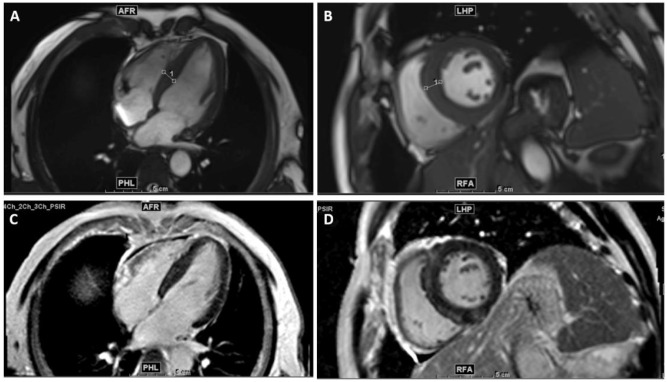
Cardiac MRI showing cine and PSIR late gadolinium enhancement (LGE) sequences. Cine bSSFP images (A, B) demonstrate asymmetric septal hypertrophy (white lines), whereas PSIR LGE images (C, D) show patchy mid-wall enhancement of the interventricular septum and inferolateral wall, consistent with an infiltrative cardiomyopathy pattern.
